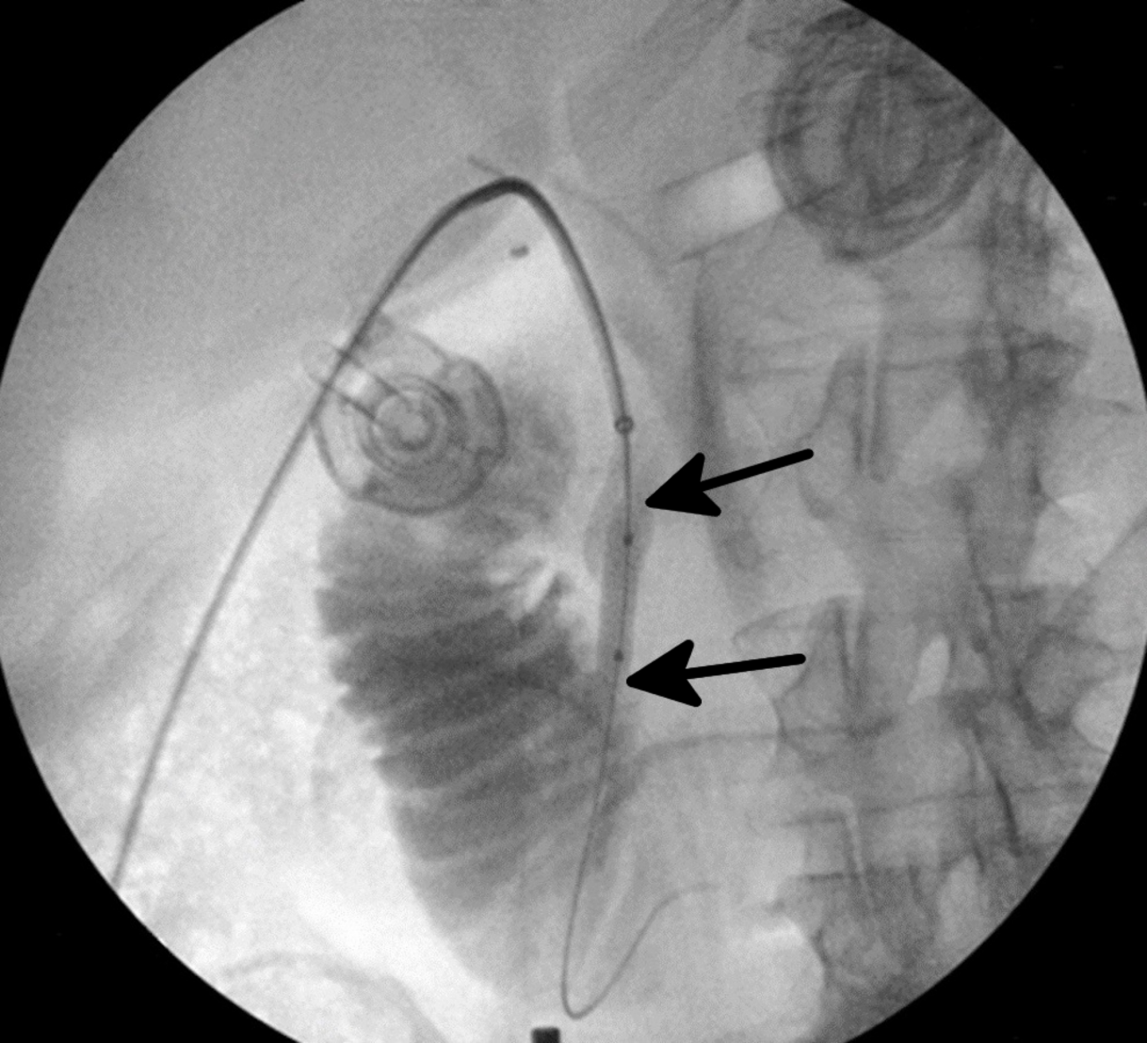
Radiographic imaging demonstrating a glidewire and TEMPO AQUA® catheter passed through the common bile duct and ampulla of Vater (black arrows). TEMPO AQUA® (Cordis, Milpitas, California, US)
